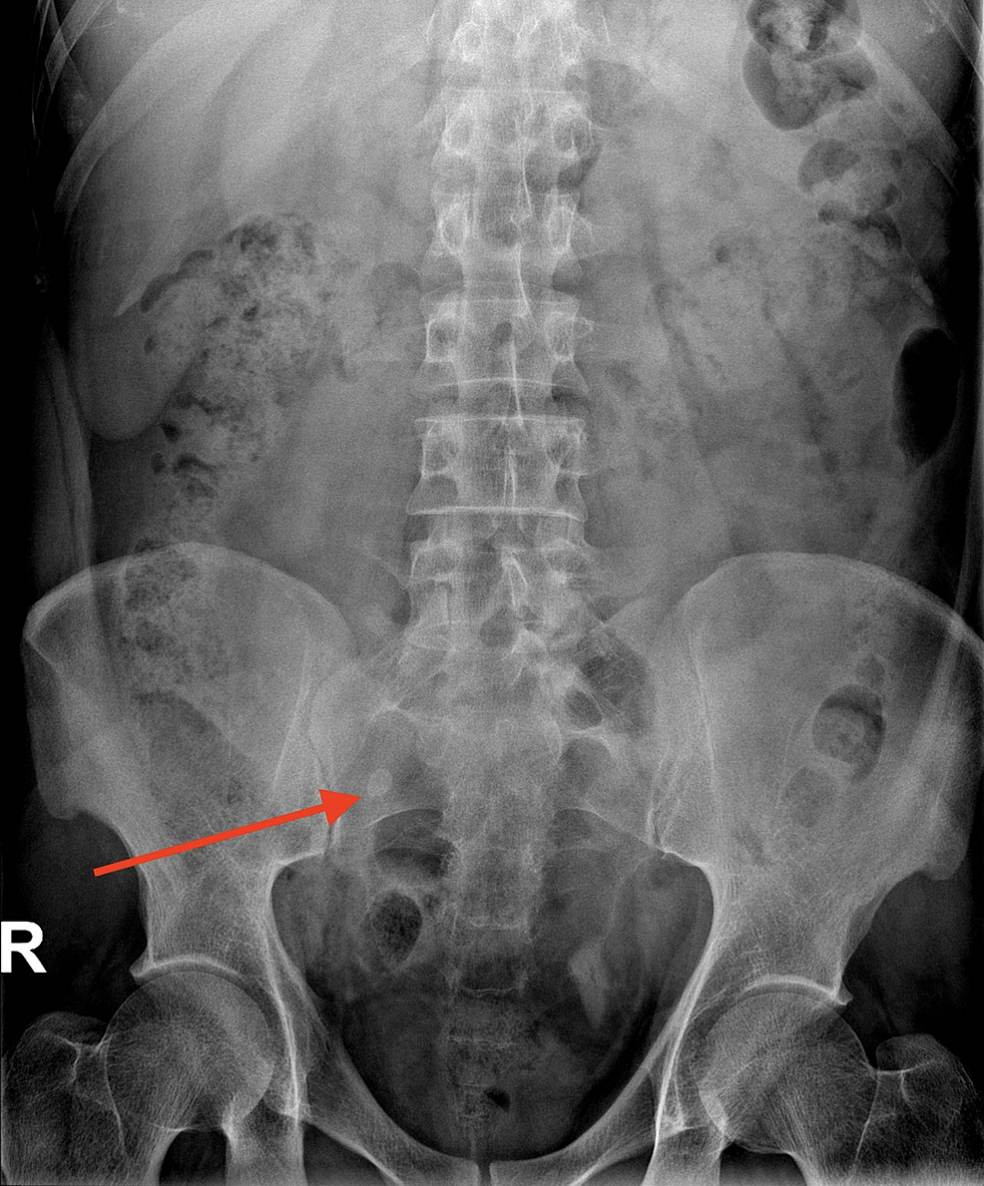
Scout image of abdomen and pelvis The scout image of the CT scan shows a well defined rounded high density structure measuring approximately 1 cm projecting over the right sacral bone in the right lower quadrant of the abdomen (red arrow).
